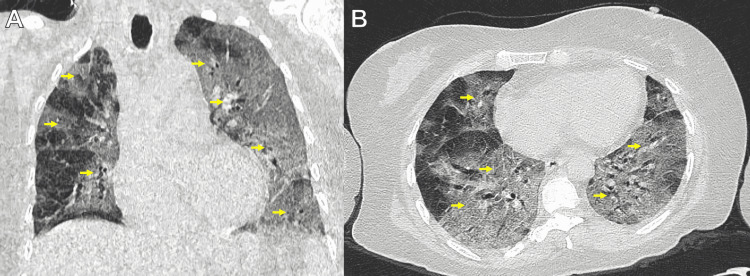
Unenhanced chest CT scan (A) Coronal and (B) axial reconstructions showing bilateral pulmonary infiltrates in all of the pulmonary parenchymas with a ground-glass pattern (yellow arrows).
